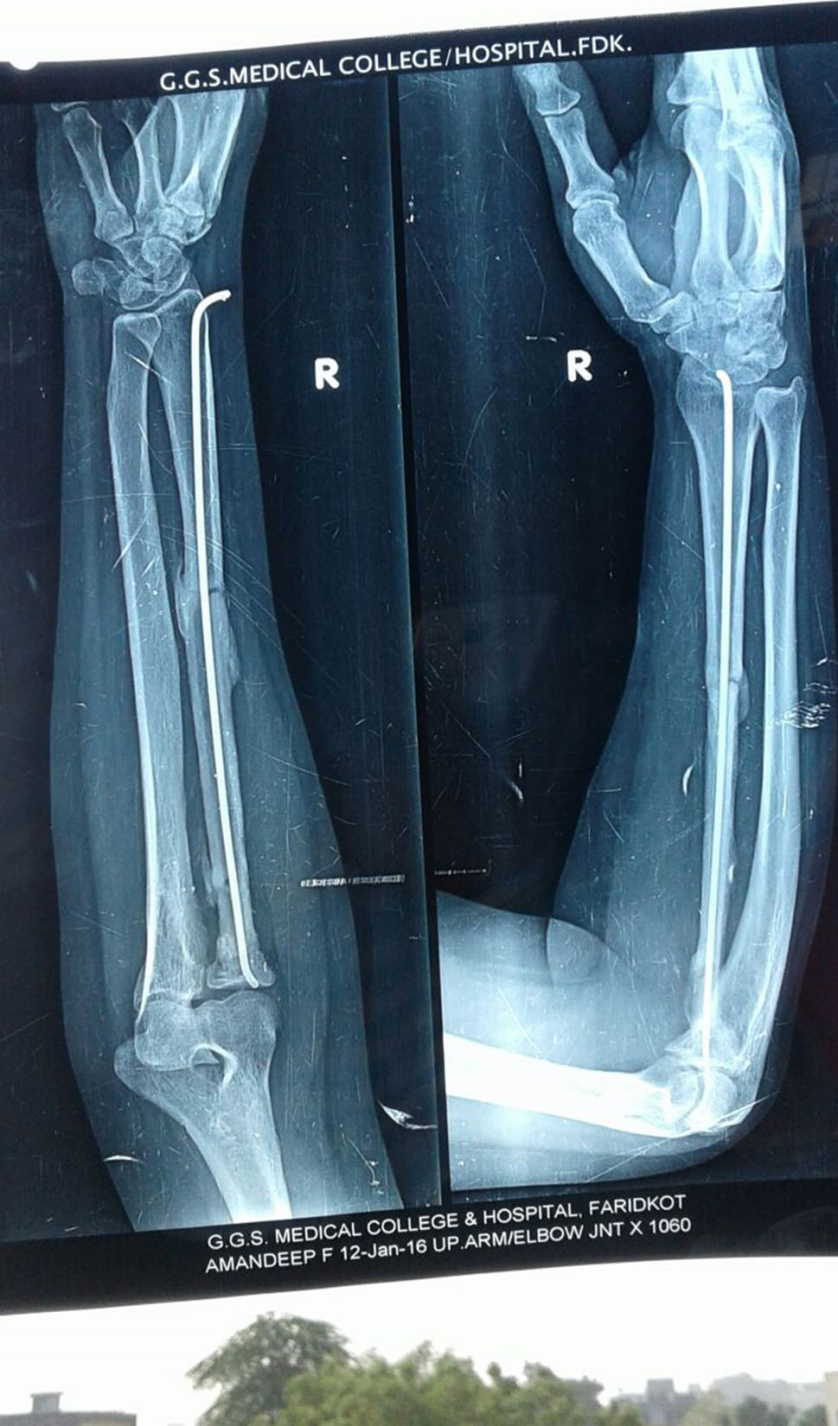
One-and-half-year-old post-operative X-ray with fibular graft along with TENS
